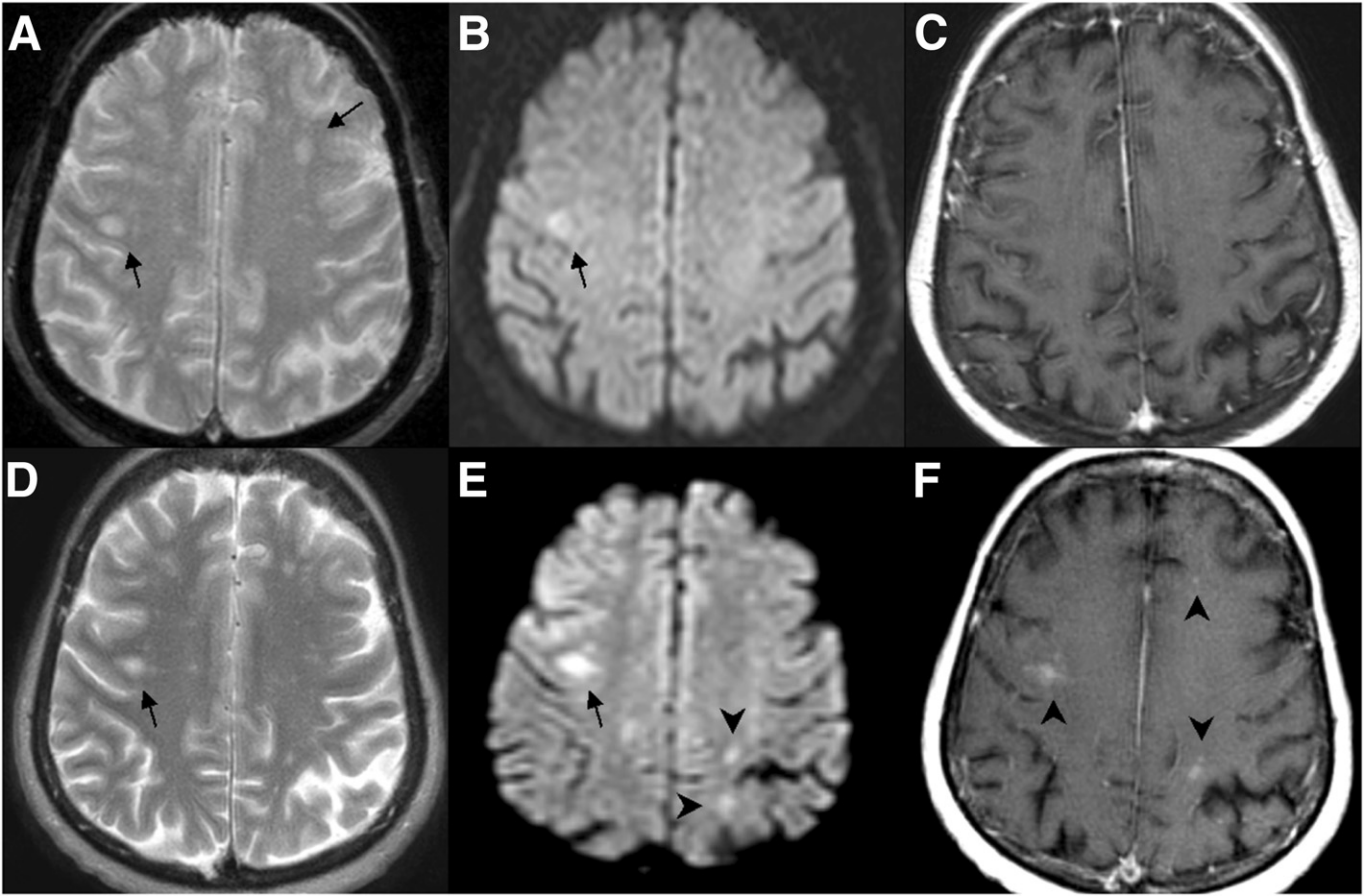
In the initial scan, T2-weighted (A) axial magnetic resonance imaging scan demonstrates small foci of disease bilaterally (arrows). The diffusion-weighted image (B) shows mild hyperintensity of the lesion on the right (arrow). The postgadolinium T1-weighted image (C) shows no significant enhancement. In the scan obtained three weeks later, the T2-weighted image (D) reveals a mild change in the lesion on the right (arrow). The diffusion-weighted image (E) reveals a marked higher signal intensity as in (B) (arrow) and shows new lesions left-sided subcortically (arrowheads). The postgadolinium T1-weighted image (F) now demonstrates marked progression of disease with patchy enhancement of lesions bilaterally (arrowheads).
